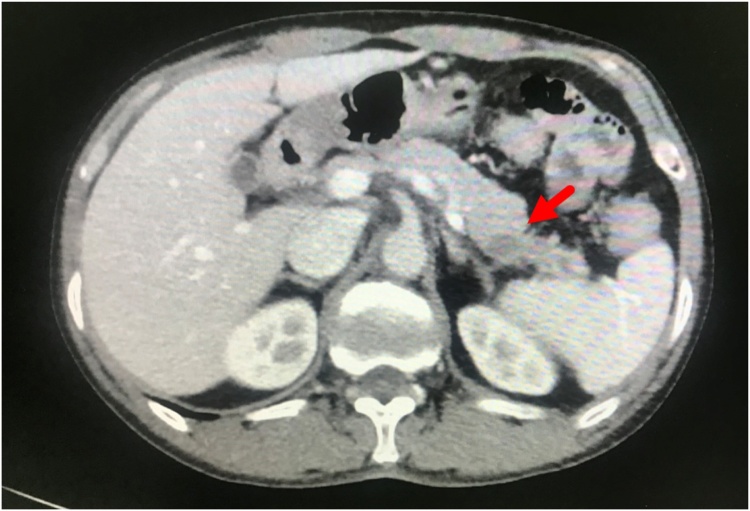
Axial view of contrasted abdominal CT showed irregular hypodense lesion at the tail of pancreas (red arrow).
